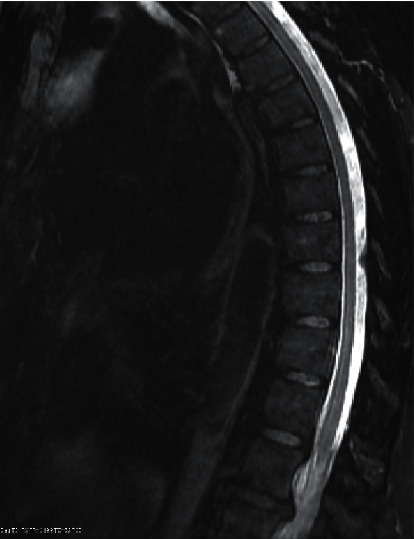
MRI with contrast, demonstrating facet spondylosis and ligamentum flavum thickening at T9-10 with mild central spinal stenosis but no cord compression. Disc degeneration at the thoracolumbar junction.
